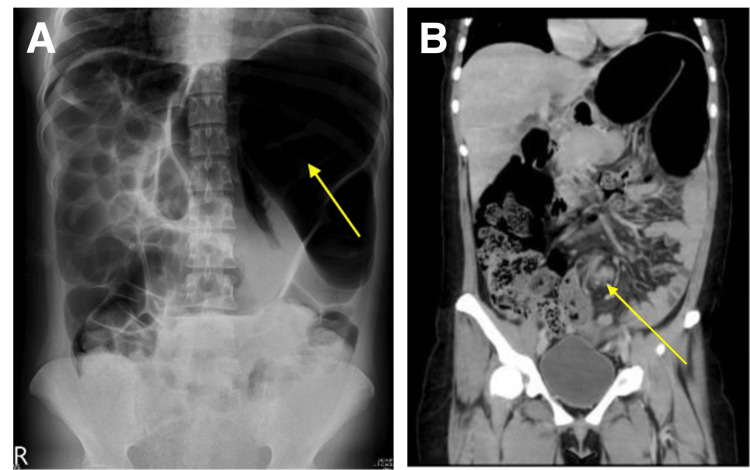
Radiographic findings of the sigmoid volvulus A: abdominal radiograph demonstrating the characteristic "reversed coffee bean sign" (yellow arrow) with a marked absence of rectal gas; B: coronal view of abdominal computed tomography revealing a dilated sigmoid colon with the pathognomonic "whirl sign" (yellow arrow), indicating torsion of the mesentery.
